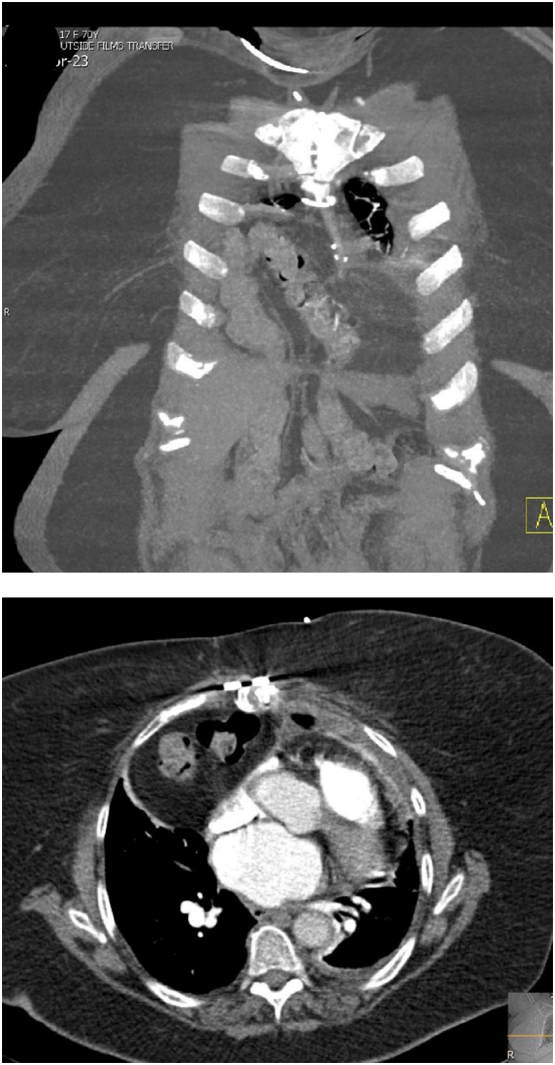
CT-scan of the chest and abdomen demonstrating a large anterior diaphragmatic hernia spanning to the anterior mediastinum and right hemithorax with herniated transverse colon and omentum.
